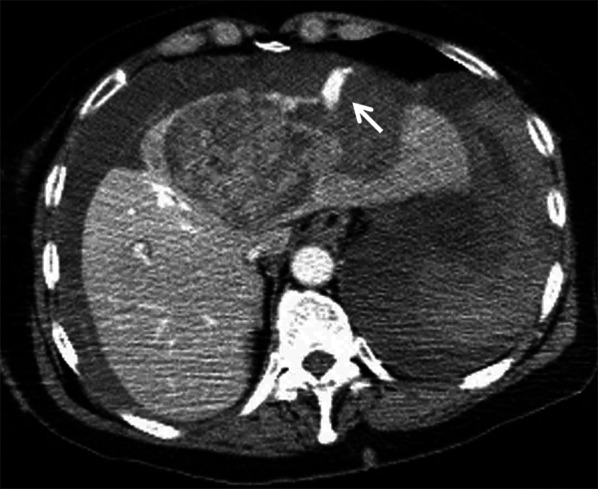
Findings of CT on arrival at the hospital. Dynamic contrast-enhanced CT scan showing extravasation of contrast medium from the ruptured tumor in the medial segment of the liver and a large amount of high-density intra-abdominal fluid collection
